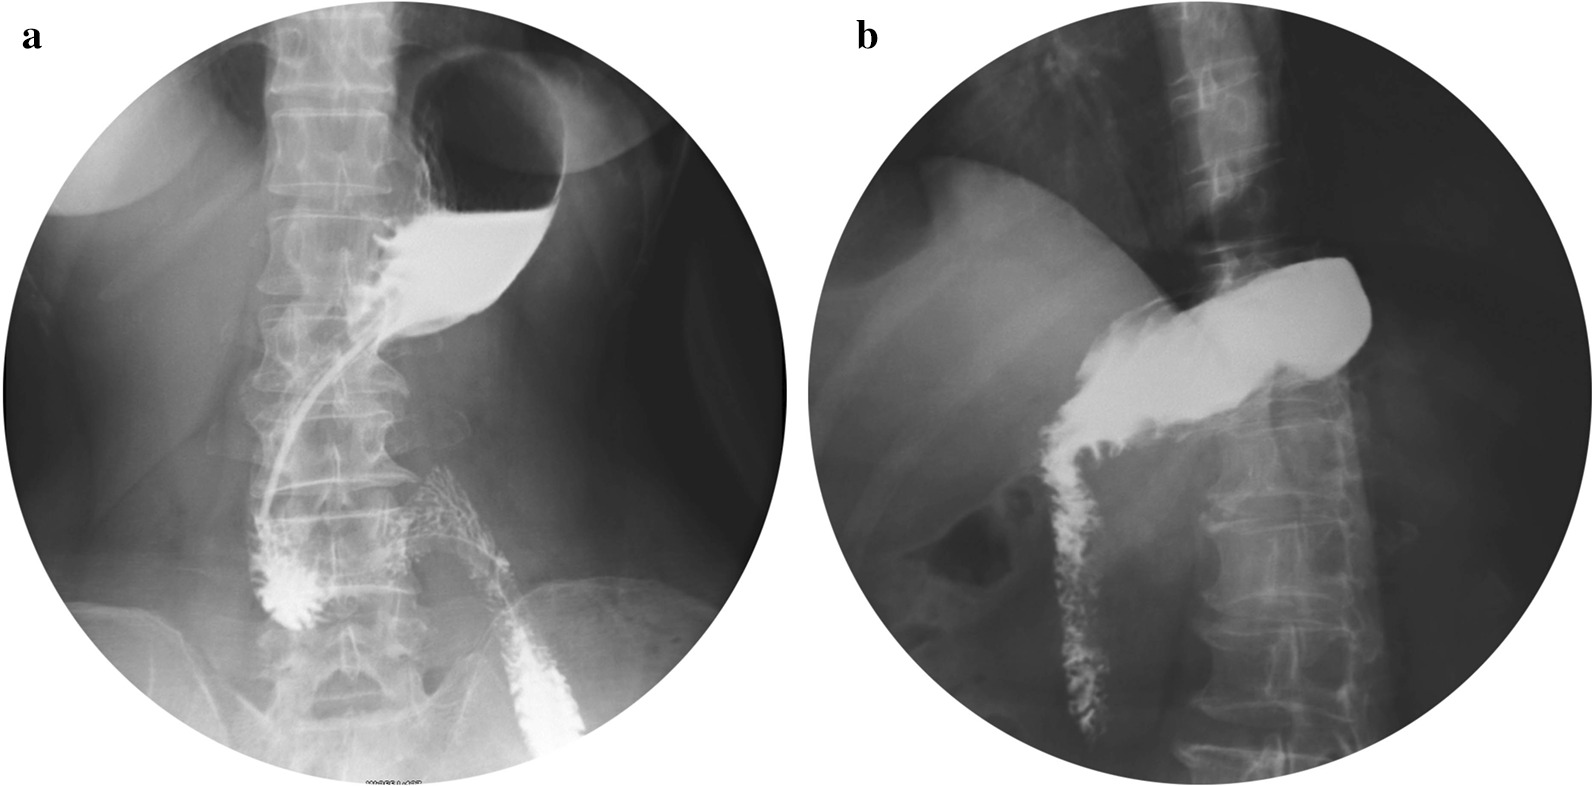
Upper gastrointestinal imaging 6 days and 1 month after operation. a X-ray performed 6 days after the operation shows that the anastomotic stoma was unobstructed and there was no anastomotic leakage. b X-ray imaging performed 1 month after the operation
